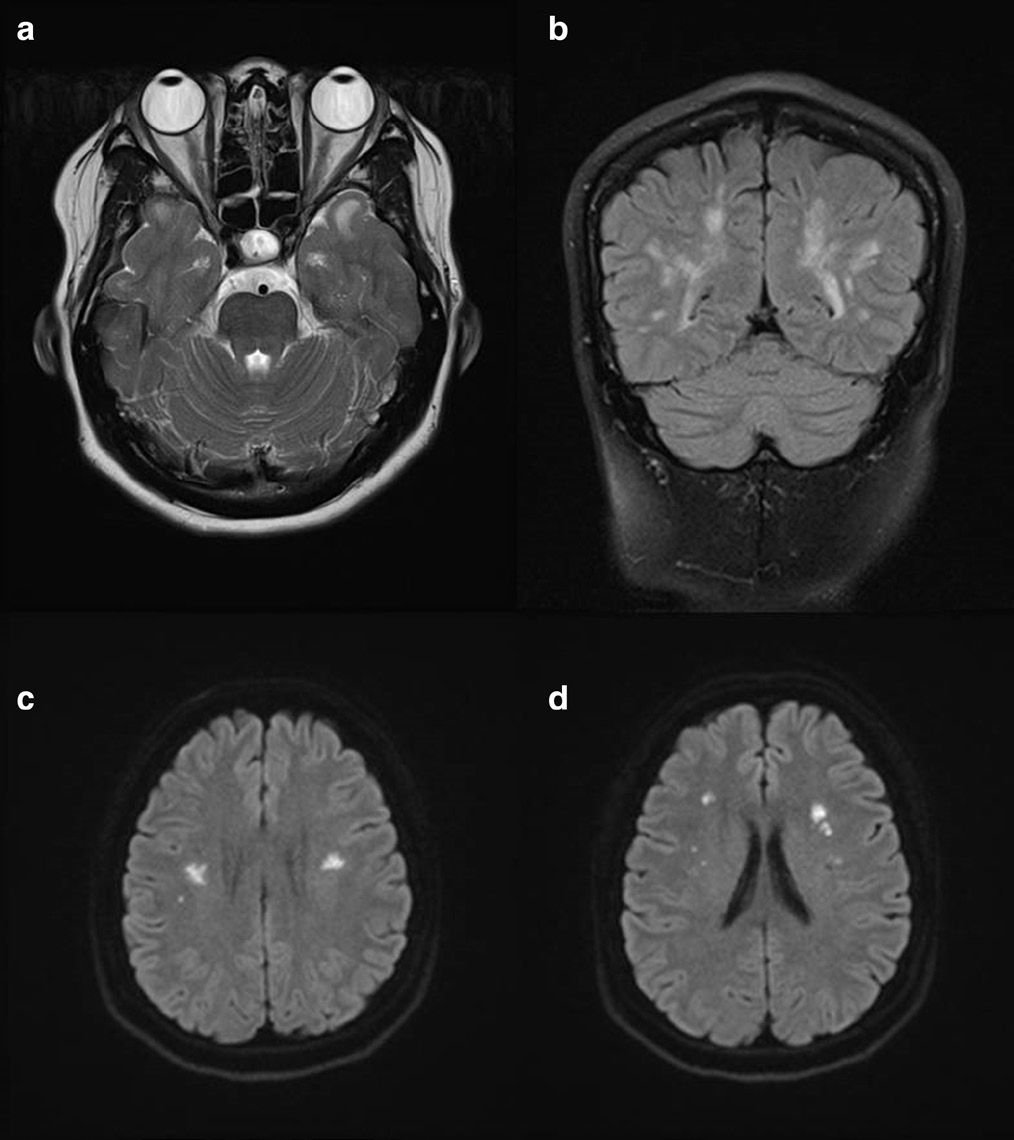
Non-contrast MRI brain axial *T_2_* weighted and coronal FLAIR MR images A) and B) Differentially involved anterior and posterior temporal white matter with symmetric distributions. Axial DWI MR images C) and D) Bilateral acute infarcts involving centrum semiovale and corona radiata.
